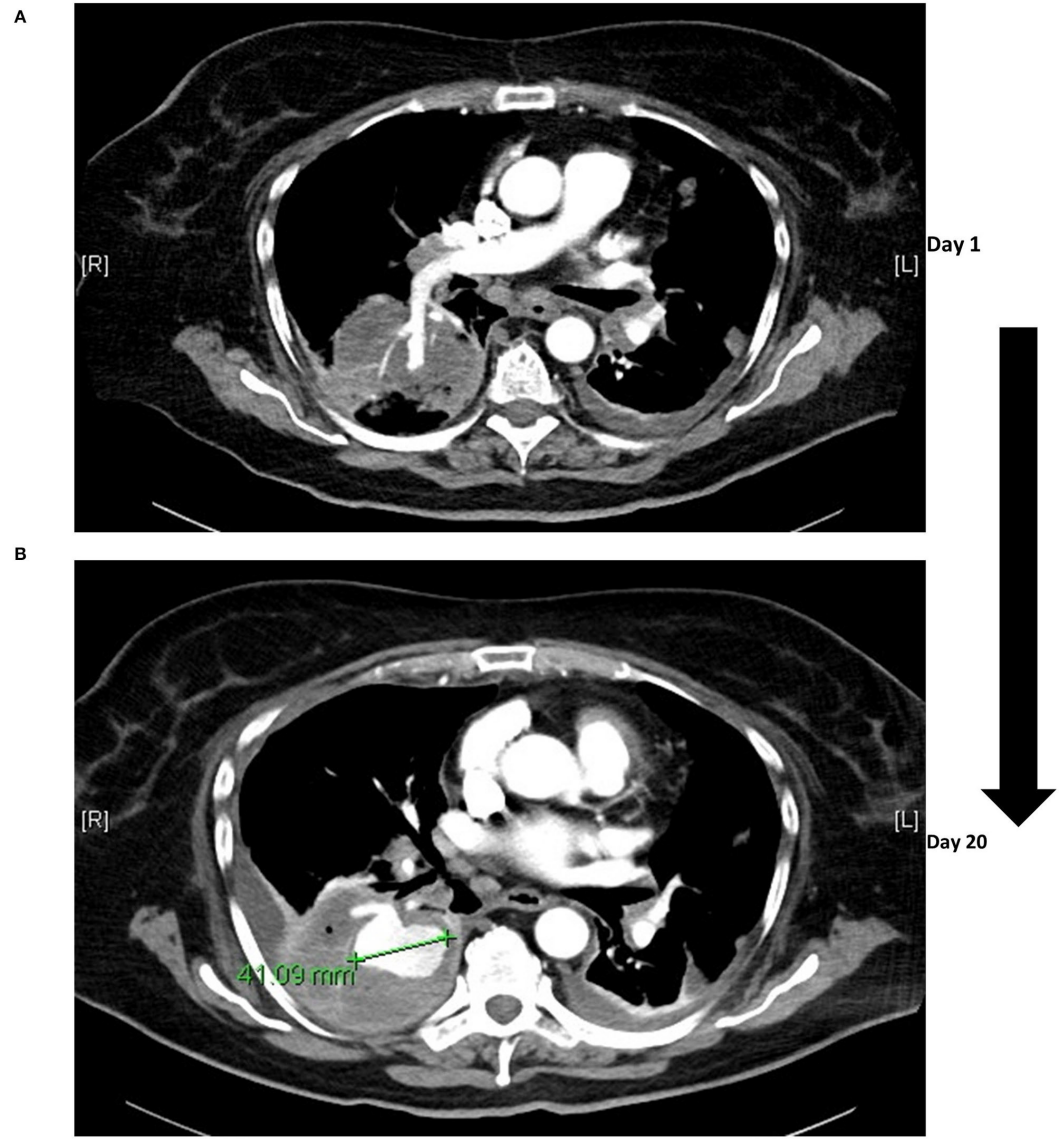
Infectious aneurysm of the pulmonary artery. The initial computed tomography was performed on the day of admission (A) with solely consolidation in the right basal lobe and an accentuated right lower lobe pulmonary artery. Over the course of 20 days an infectious pulmonary arterial aneurysm manifested with a diameter >4 cm (B), coinciding with clinical signs of hemoptysis.
